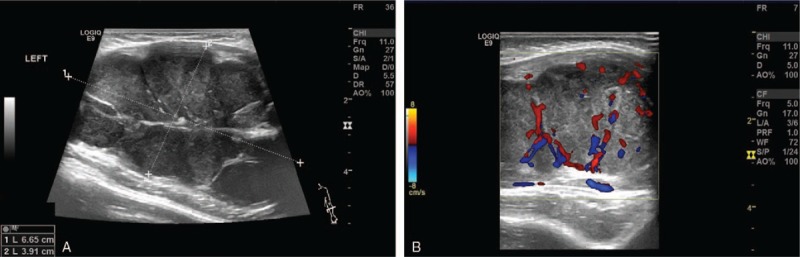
B-scan ultrasonography a 7.8 cm × 3.8 cm × 4.3 cm hypoechoic mass in the muscle layer with clear boundary and lobulated shape; Color Doppler flow imaging (CDFI) showed abundant blood flow signals in the mass.
